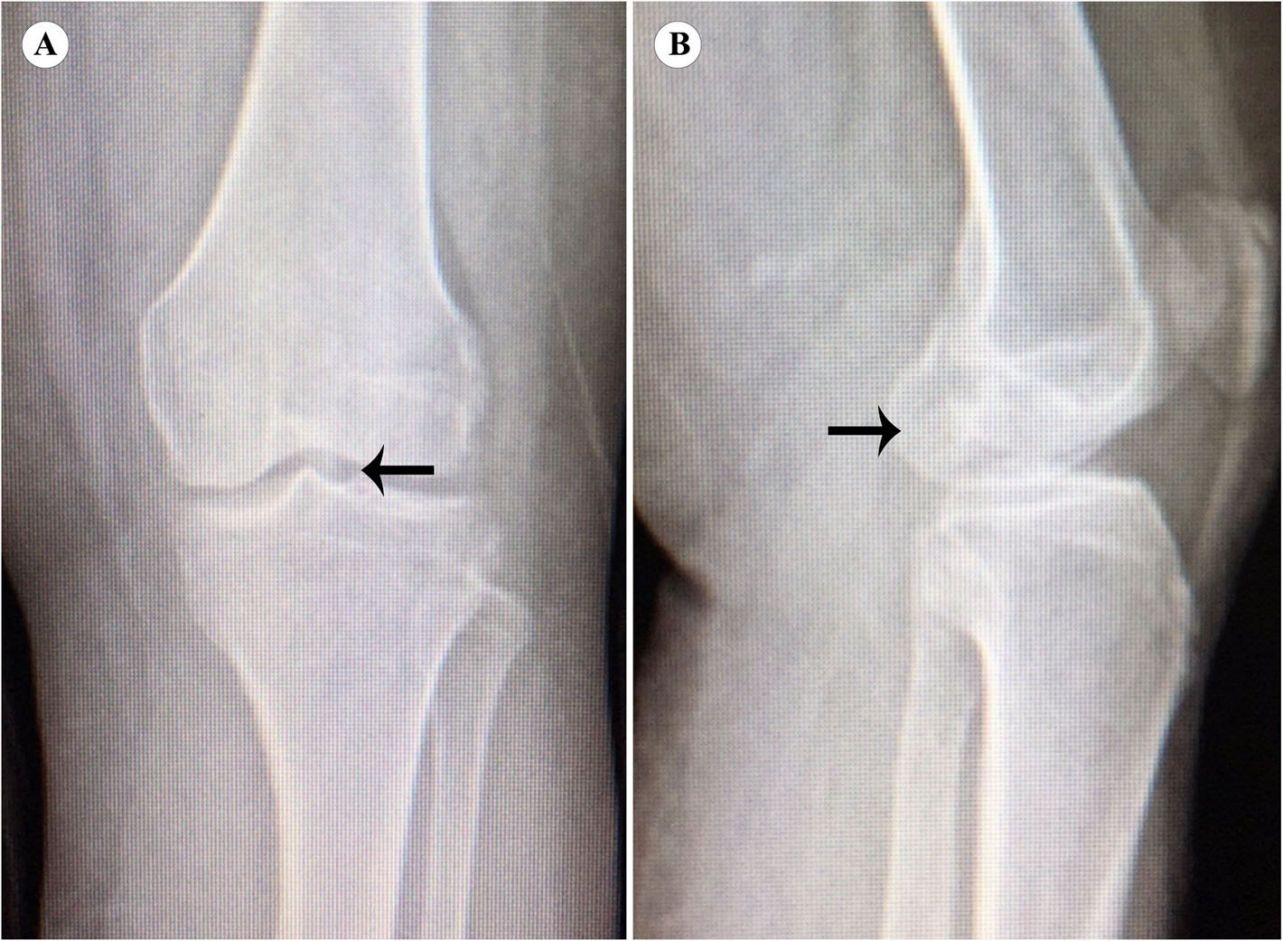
A dimly glimpsed high-density mass within the intercondylar notch is showed on X-ray of the left knee (arrow)
